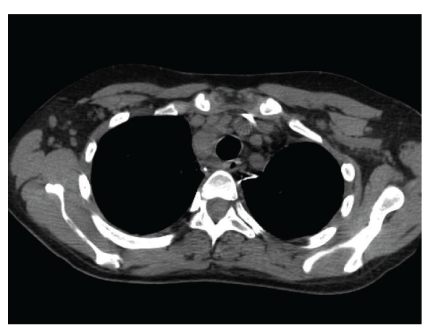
CT scan of chest demonstrated multicompartmental mediastinal lymphadenopathy, for example, a right paratracheal node measuring 14 mm in short axis.
